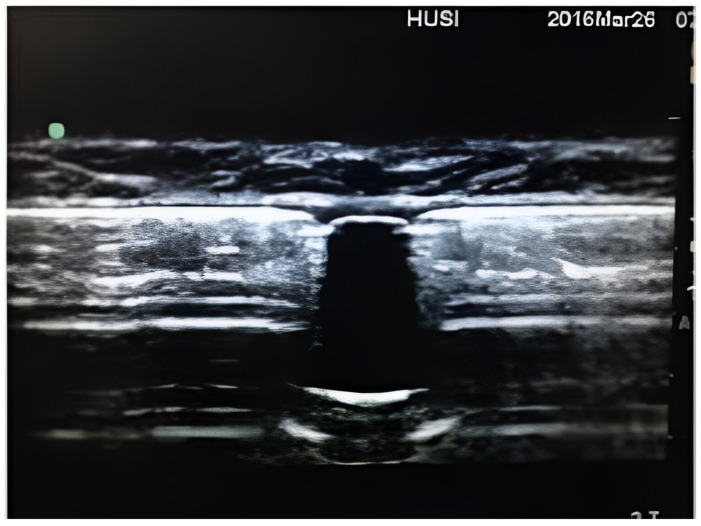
Refill port as seen with the ultrasound device.
